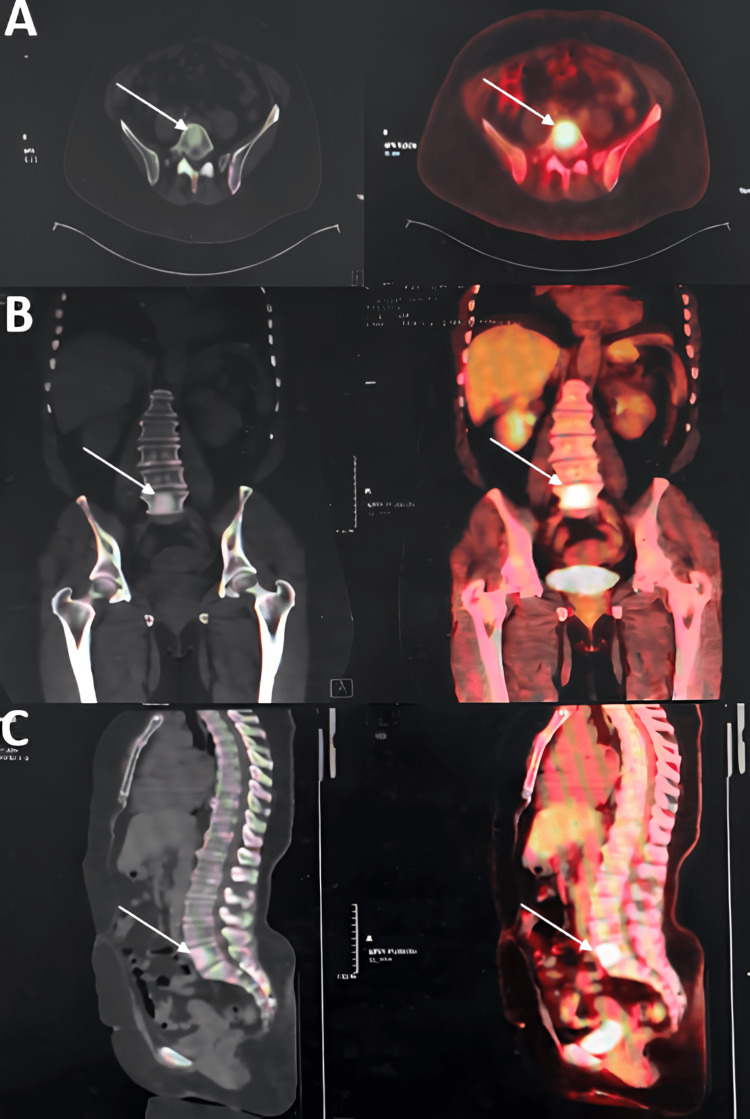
PET-CT images of the L5 vertebra Axial (A), coronal (B), and sagittal (C) sections show an osteocondensing and hypermetabolic lesion (white arrow) of the L5 vertebral body of secondary origin.
